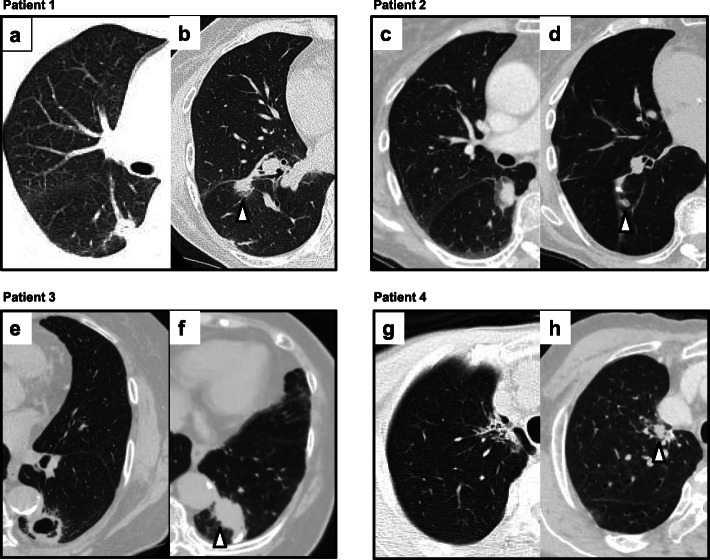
CT findings in the four patients. a Primary lesion in the right superior segment in patient 1; 15 mm in diameter. b Recurrent lesion (37 months after segmentectomy) on the staple line in patient 1 (arrowhead); 20 mm. c Primary lesion in the right superior segment in patient 2 (27 mm). d Recurrent lesion (39 months after segmentectomy) on the staple line in patient 2 (arrowhead) (10 mm). e Primary lesion in the left superior segment in patient 3 (28 mm). f Recurrent lesion (41 months after segmentectomy) on the staple line in patient 3 (arrowhead) (45 mm). g Primary lesion in the right apical segment in patient 4 (35 mm). h Recurrent lesion (16 months after segmentectomy) on the staple line in patient 4 (arrowhead) (10 mm)
